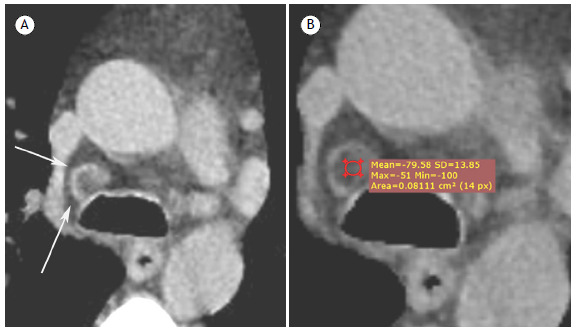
Detail of an axial CT scan of the chest, showing a mediastinal lymph node of normal dimensions (arrows), with a hypodense center (mean density = −80 HU) and a halo with greater, homogeneously thick density on the periphery.
